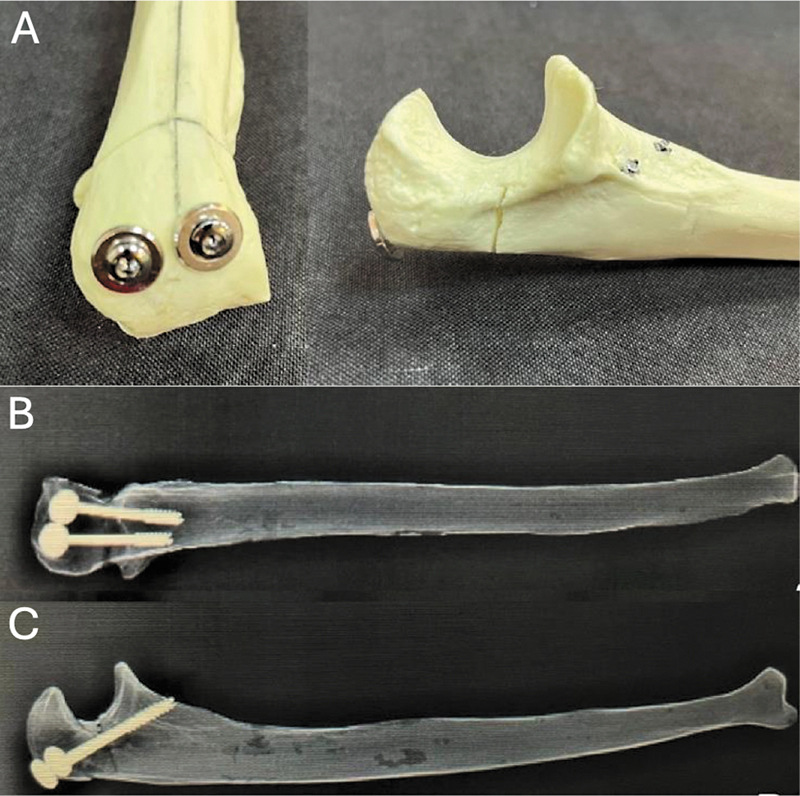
Transcortical screw (TC) fixation. (A) Osteotomy fixation by perpendicular insertion of two TC screws. (B) Anteroposterior and lateral (C) radiographs of radiopaque ulnae after osteotomy fixation with TC screws.
